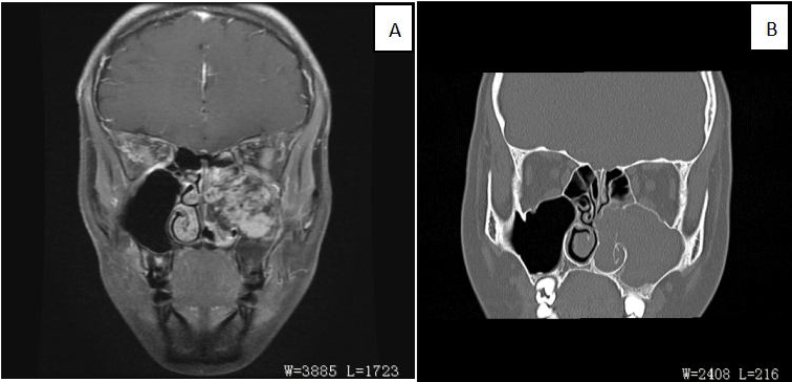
(A) Coronal fat-suppressed contrast-enhanced T1WI showed that the soft tissue mass in the left maxillary sinus displayed uneven and obvious enhancement (red arrow). Bone was discontinuous in medial wall, posterior wall and parietal wall of maxillary sinus. (B) The coronal CT bone window reconstruction image showed an expansion of the left maxillarysinus, increased density in the sinus cavity, and the lesion through the sinus opening into the left nasal cavity. The bone of the left middle and lower turbinates was resorpted, and the boundary with the lesion was unclear.
